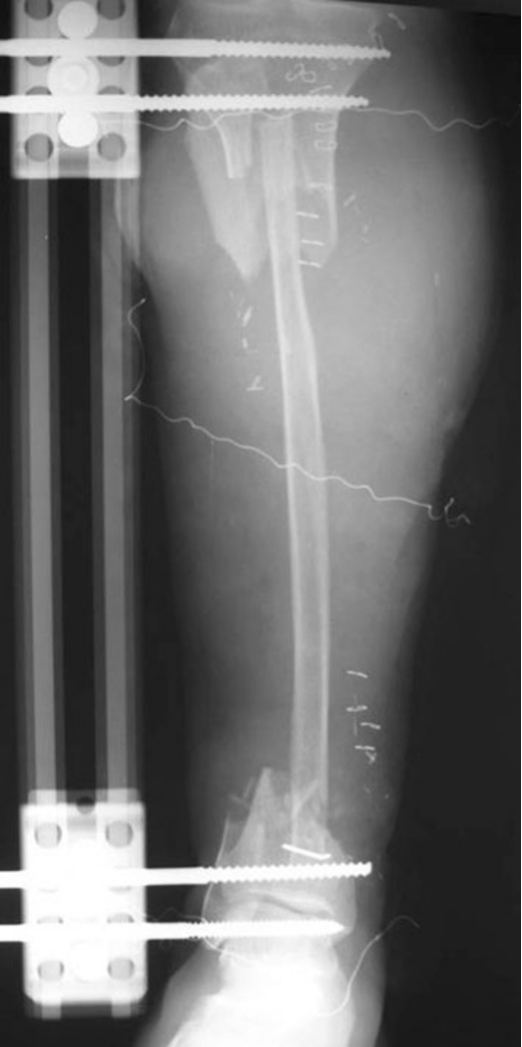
An early postoperative radiograph shows that the fibula is seated well between the two poles of the tibia
